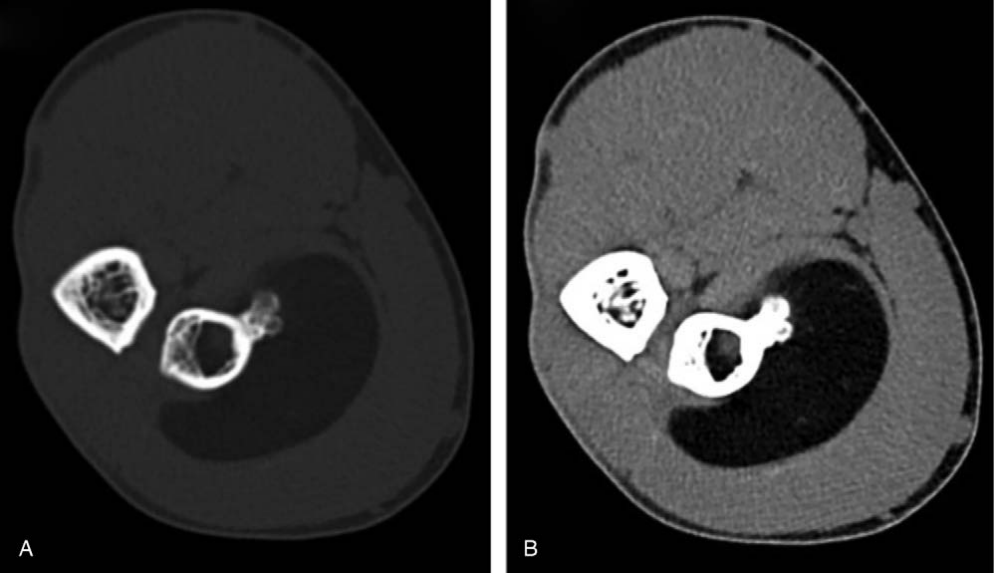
Parosteal lipoma of the proximal anterolateral forearm with internal radial bone protuberance. Axial bone window (A) and soft tissue window (B) computed tomography scan of the right forearm.
